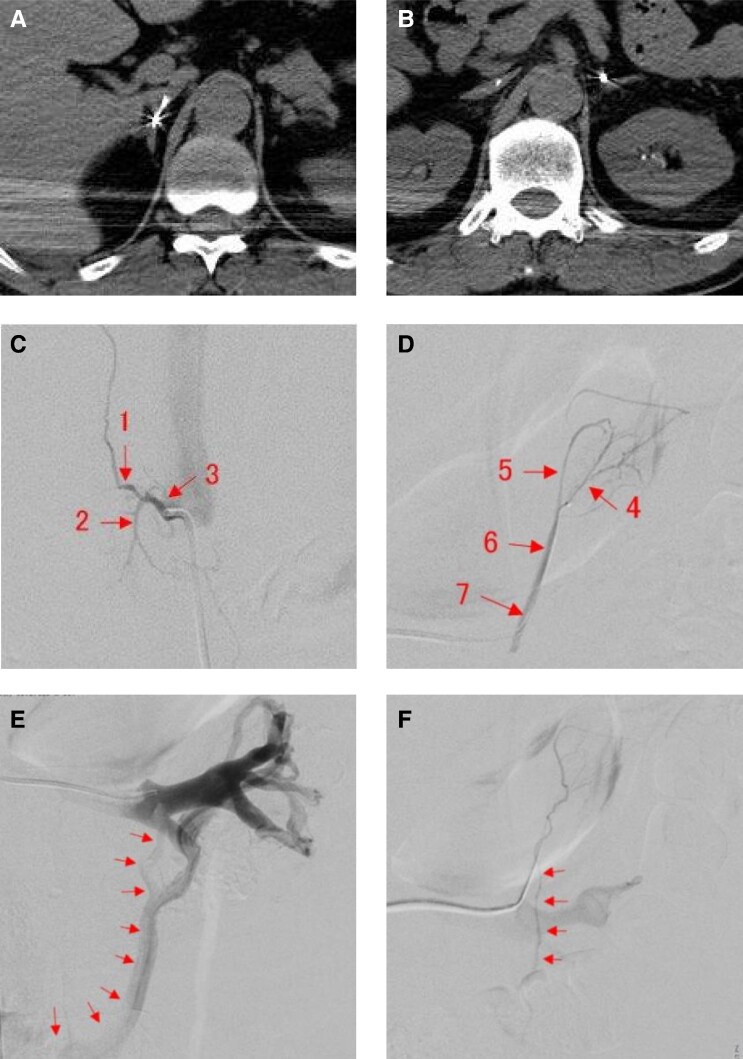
CT angiographic images from the second S-AVS. (A) Guidewire insertion into the right adrenal vein. (B) Guidewire insertion into the left adrenal vein. (C) 1: upper tributary; 2: lower tributary; 3: central vein of the right adrenal vein. (D) 4: superolateral tributary; 5: superomedial tributary; 6: common trunk; 7: central vein of the right adrenal vein. (E) Contrast angiography of the left renal vein showing the circumaortic vein draining into the inferior vena cava. (F) An inferior emissary vein seen in contrast from branch 4 was suspected to flow into this vessel. Abbreviations: CT, computed tomography; S-AVS, segmental adrenal vein sampling.
